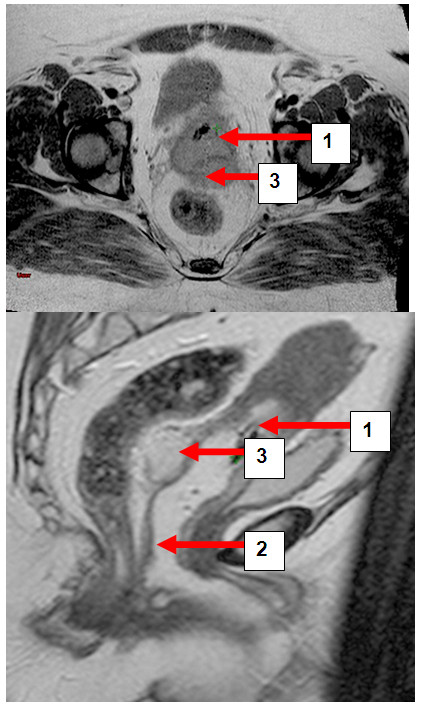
a and b: Patient MB. Transversal and sagittal pelvic MRI (T2-FSE) with the cervical carcinoma (1) vaginal gel (2), rectal separation (3) and afterloading applicator in situ (4).
